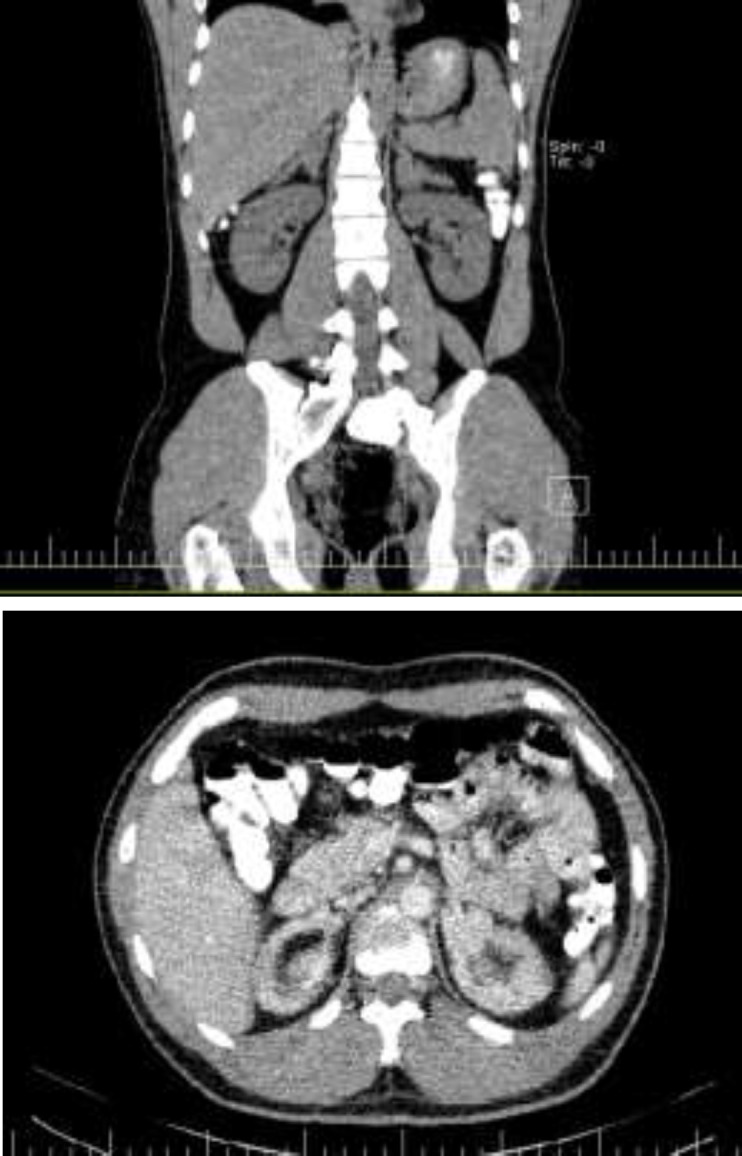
CT scan imaging of patient before left orchiectomy in coronal (a) and transverse (b) sections
